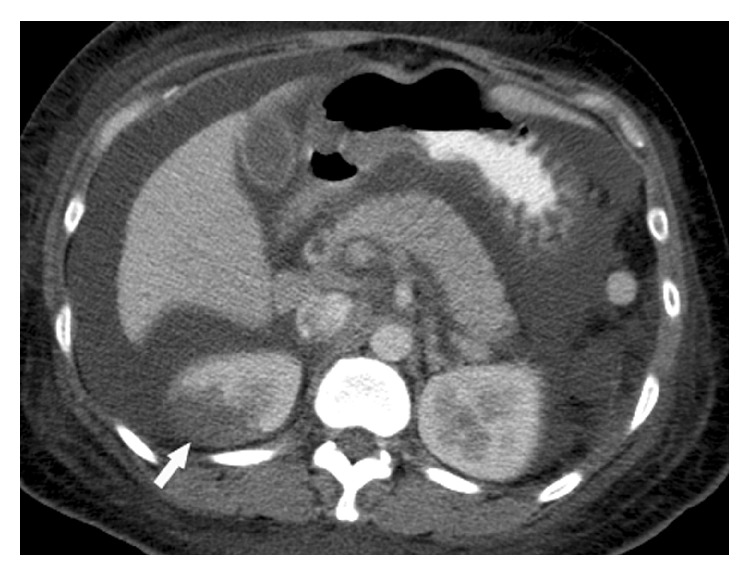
The axial contrast-enhanced CT image showing a wedge-shaped infarction (arrow) involving the right kidney.
